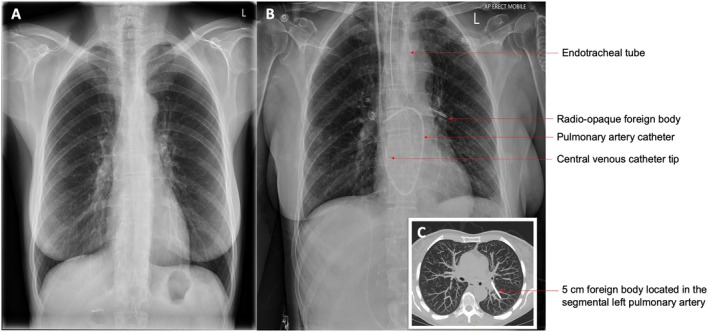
Preoperative (A) and postoperative (B) chest radiographs showing new linear opacity near the left hilar region, which was confirmed on chest computed tomography to be located within a segmental branch of the left lower lobe (C).
